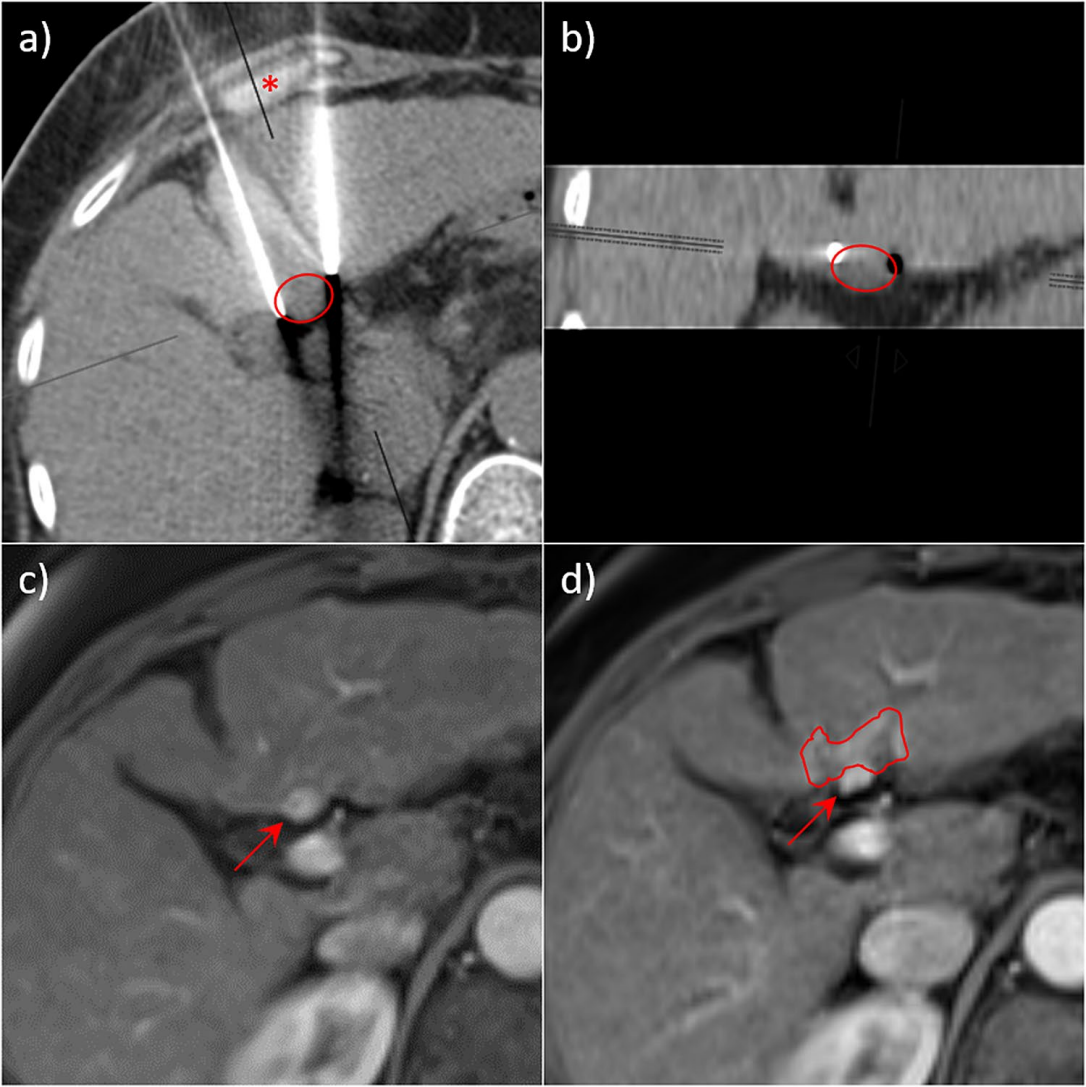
Example of an IRE with residual tumor after ablation with 2 needles. (a) and (b) show multi-planar reconstructions (MPRs) of the computed tomography (CT) with needle placement immediately before ablation of a hepatocellular carcinoma (HCC), (a) in parallel alignment to the needle plane, (b) orthogonal to it. The tumor location is outlined as red circle. Here 1. the needle depth was insufficient (Ndep = 11 mm) due to the subcapsular location of the tumor, 2. the needle divergence was high (Ndiv = 8.5°) because of the unfavourable interposition of the rib cartilage (asterix) and 3. the tumor was slightly caudal to the needle plane. This results in a high tumor-center-to-ablation-center distance (TACD = 10 mm). (c) Contrast enhanced magnetic resonance imaging (MRI) before ablation showing the hypervascularised HCC (arrow). (d) residual tumor 6 weeks after ablation. The distorted ablation area, which spares the tumor area, is outlined in red.
